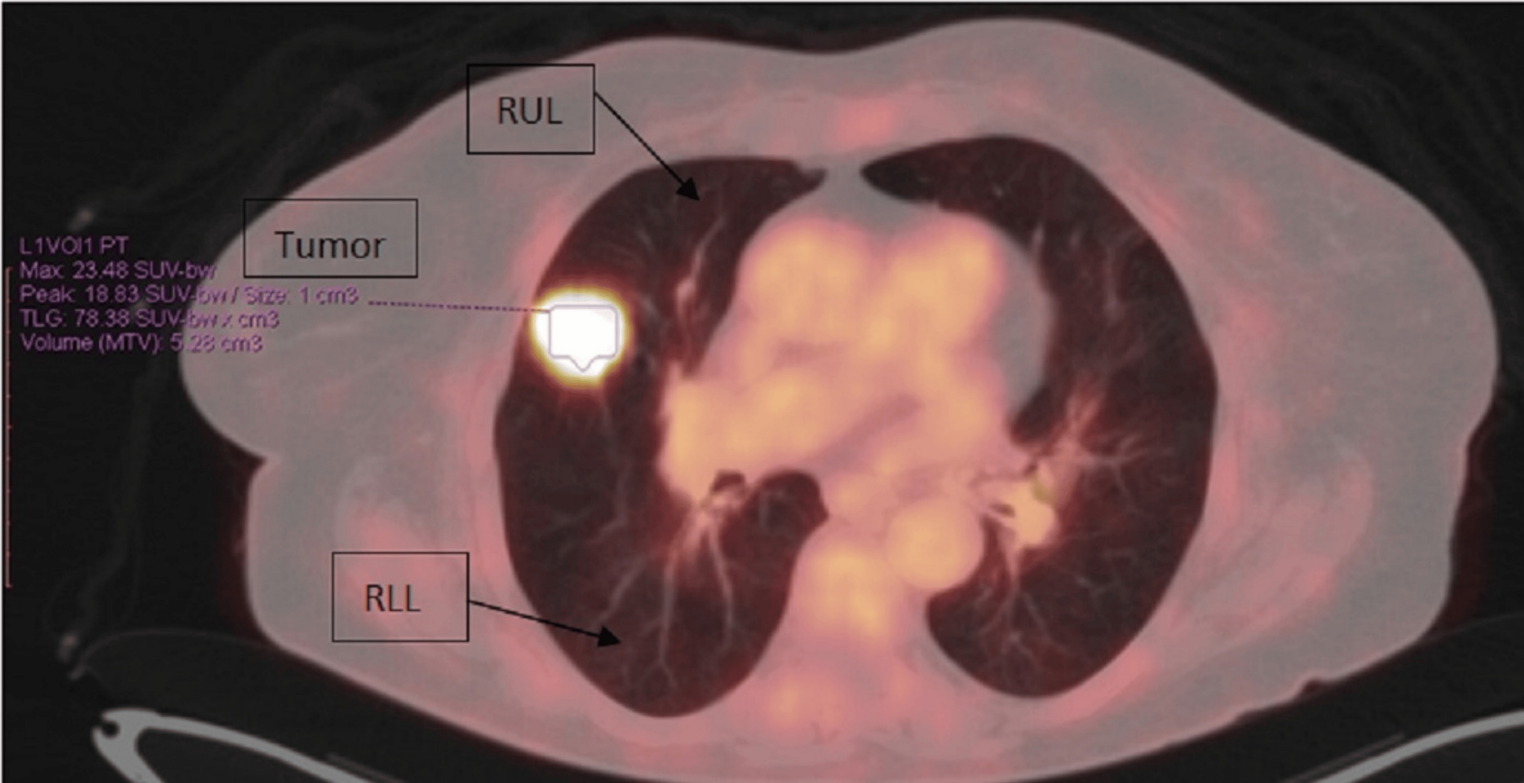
The PET-CT scan showed increased metabolic uptake in the right upper lobe nodule without evidence of hypermetabolic activity in the lymph nodes. RUL: right upper lobe; RLL: right lower lobe
